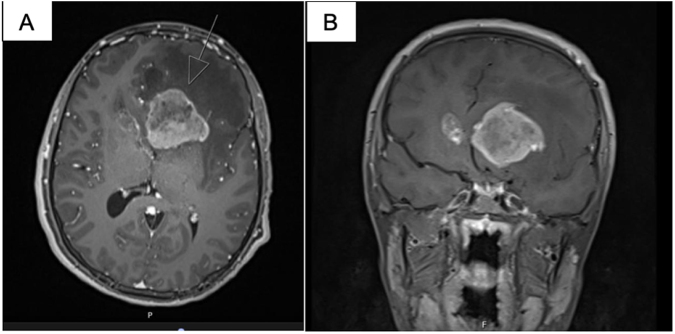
T1-weighted MRI showing a periventricular mass (41 × 32 × 13 mm) near the left frontal horn with an associated contralateral nodule in the caudate head (14 × 13 x 17 mm), and a 6 mm nodule in the right cerebellar hemisphere (a, axial; b, coronal).
